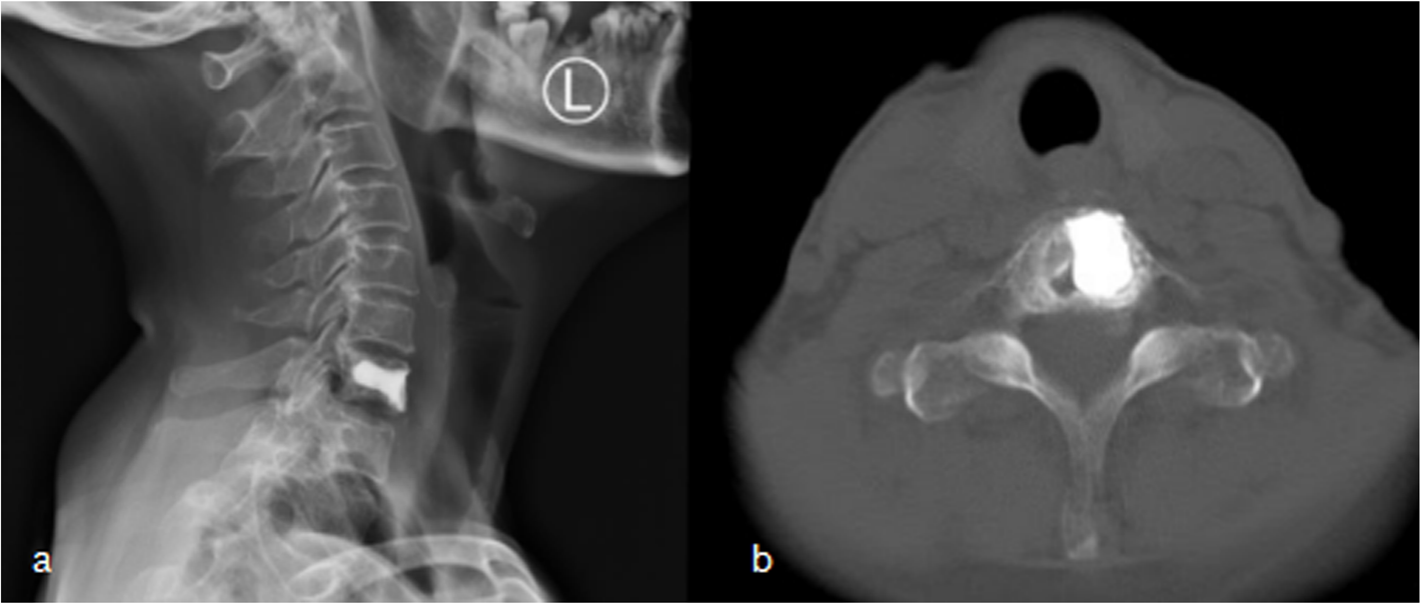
Twelve months after surgery imaging of X-ray (a) and CT (b)
